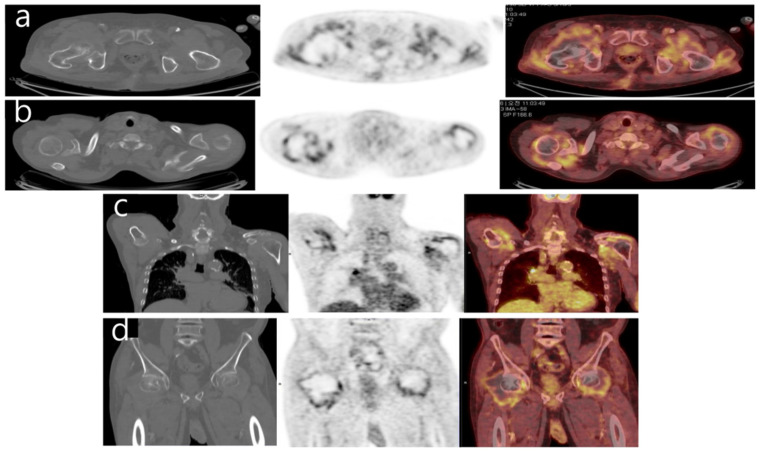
^18^F-FDG PET-CT axial (a,b) and coronal (c,d) images of both shoulders and hips demonstrate diffuse periarticular uptake. Non-contrast CT obtained in conjunction with PET-CT imaging showed osseous erosions involve both humeral and femoral heads.
